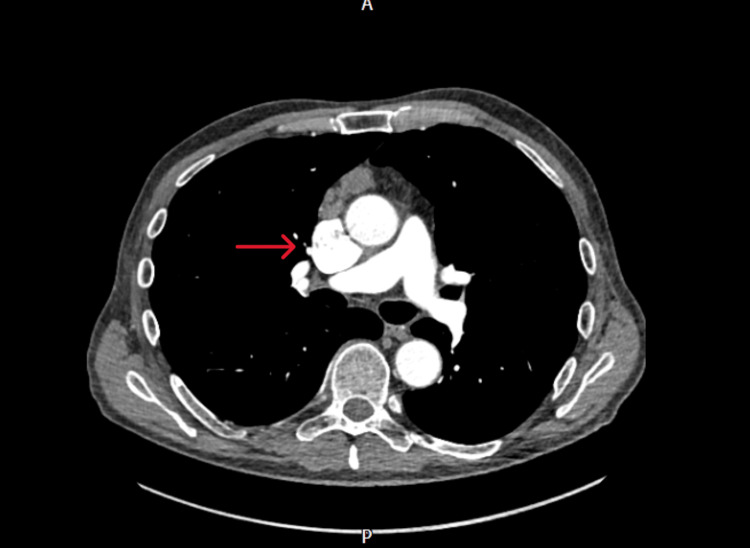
Contrast-enhanced computed tomography image 1 (axial view) The image is showing the large fusiform superior vena cava aneurysm (red arrow).
